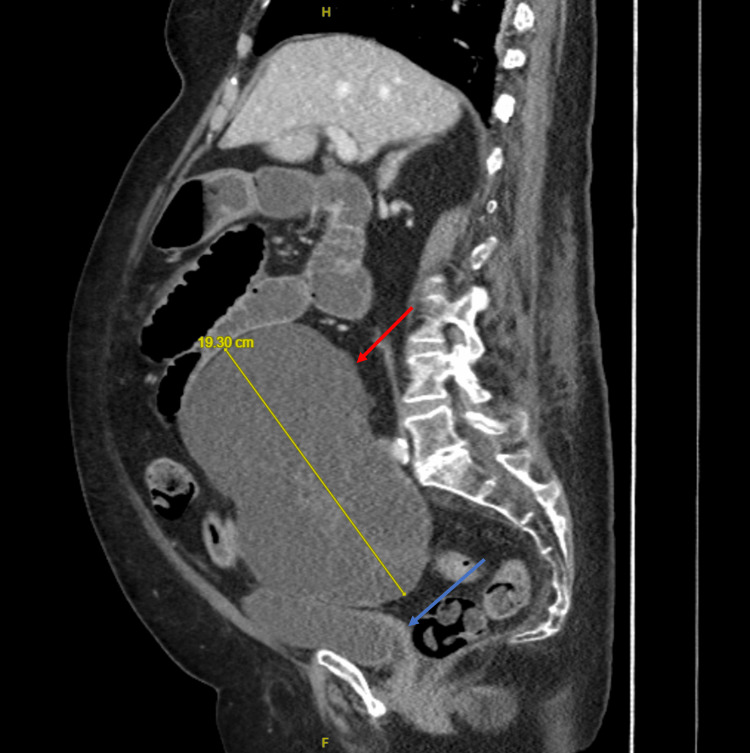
Contrast-enhanced CT scan in the sagittal view reveals a large cystic mass (red arrow) measuring 19.3 cm craniocaudally. The compressed urinary bladder (blue arrow).
